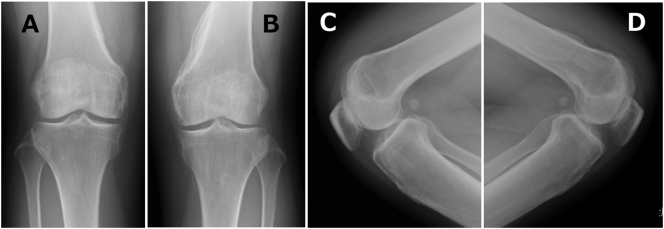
Postoperative anteroposterior and lateral radiographs of both knees showing no recurrence of the ossific mass 36 months post-excision and no change in passive range of motion. A: anteroposterior radiographs of the right knees B: anteroposterior radiographs of the left knees C: lateral radiographs of the right knees D: lateral radiographs of the left knees.
